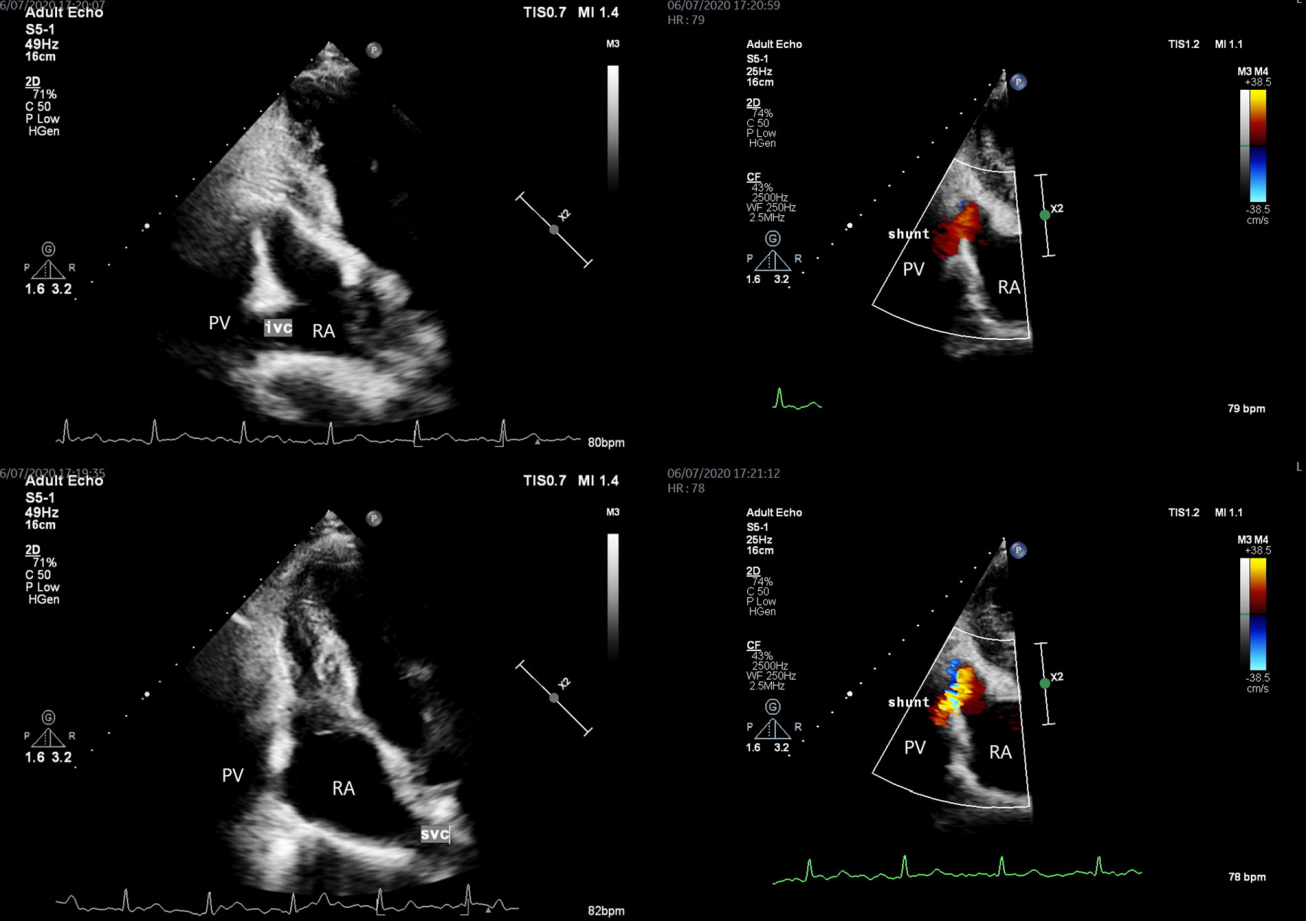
Subcostal transthoracic echocardiography imaging demonstrating the inferior vena cava (ivc), superior vena cava (svc), and extrahepatic portosystemic shunt (shunt) – Color doppler demonstrates venous flow diversion from the portal vein (PV) into the right atrium (top and bottom right)
